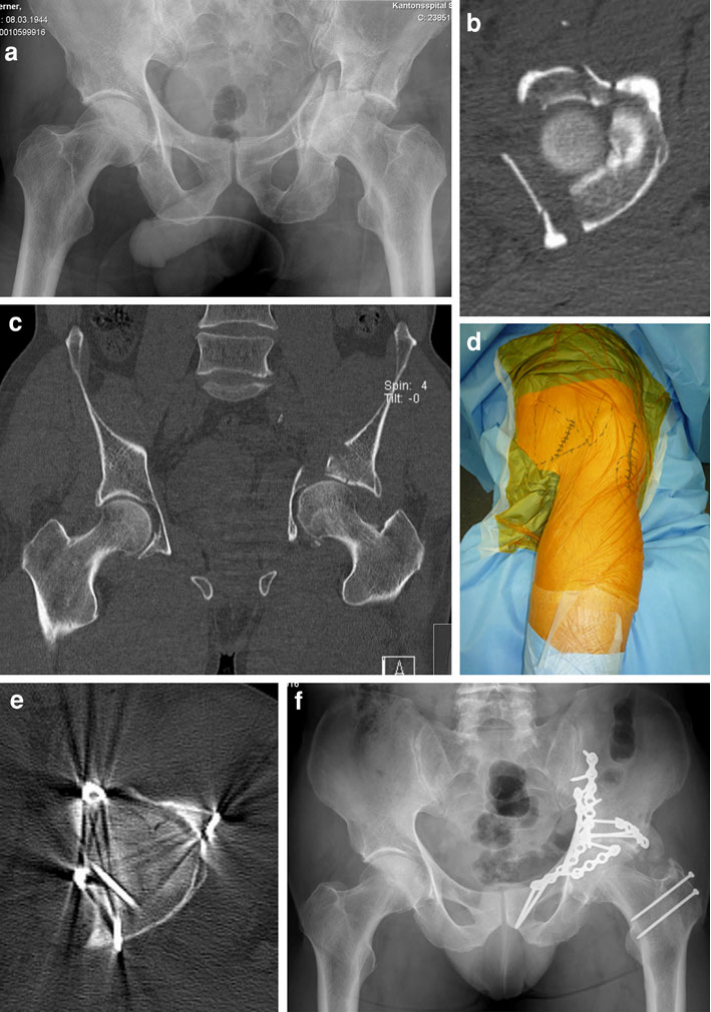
a Anteroposterior radiograph of the pelvis of a 67-year-old patient following a bicycle accident that caused a both-column acetabular fracture with central femoral head dislocation, a medialized quadrilateral plate, and dome impression. b Axial CT scan of the pelvis demonstrates a both-column acetabular fracture with central femoral head dislocation, a medialized quadrilateral plate, and dome impression. c Coronal CT scan of the pelvis demonstrates a both-column acetabular fracture with central femoral head dislocation, a medialized quadrilateral plate, and dome impression. d Intraoperative view of the floppy semilateral position that allows simultaneous anterior access by the Pararectus approach and posterior access by the trochanteric flip osteotomy and surgical hip dislocation. e Postoperative axial CT scan showing anatomic reconstruction of the left hip. f Anteroposterior radiograph of the pelvis 1 year after surgery, with some ectopic ossifications present posteriorly (Brooker grade 2)
